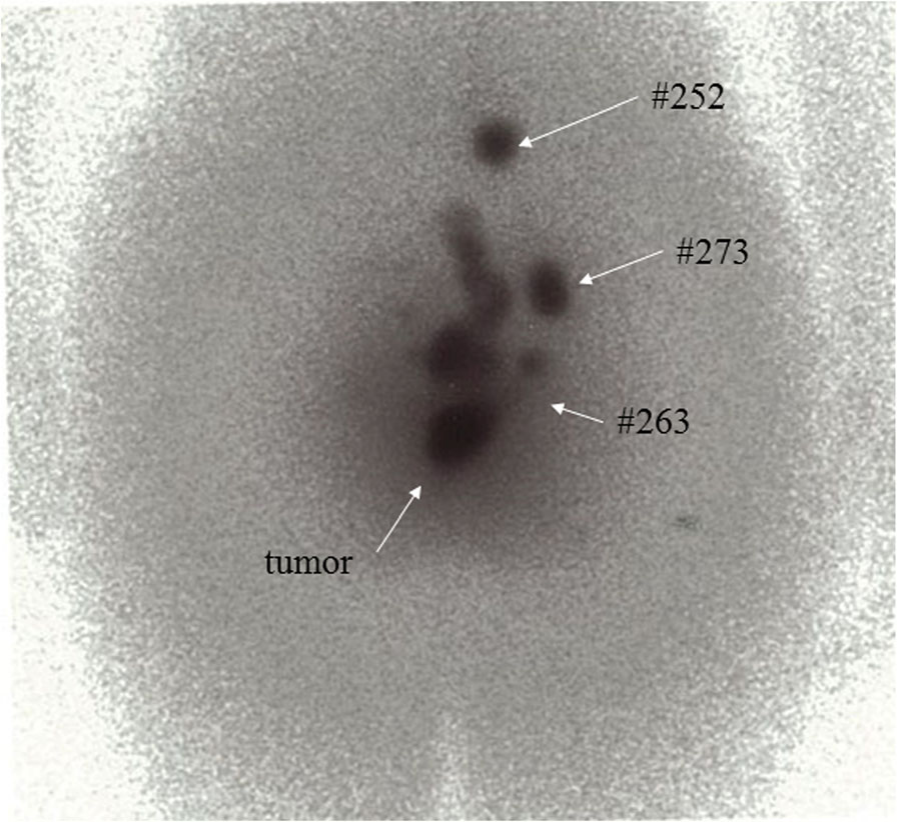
Preoperative lymphoscintigraphy after endoscopic injection of radio isotope. HNs in lateral pelvic lymph nodes are indicated as allows
